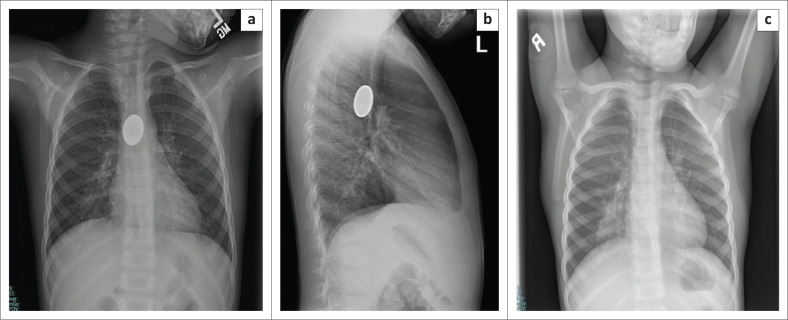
Initial frontal (a) and lateral (b) chest radiographs demonstrate a coin in the mid oesophagus of a 4-year-old male child. A follow-up radiograph (c) indicates that the coin has passed into the stomach. The child was managed conservatively.
